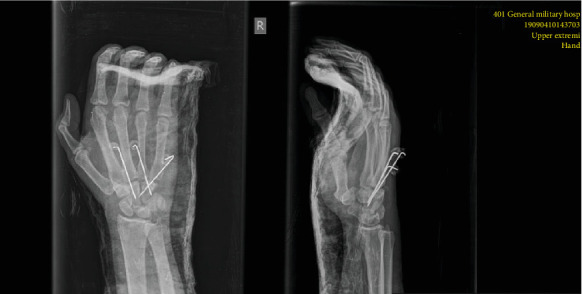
Radiographs after open reduction and fixation with Kirschner wires.
